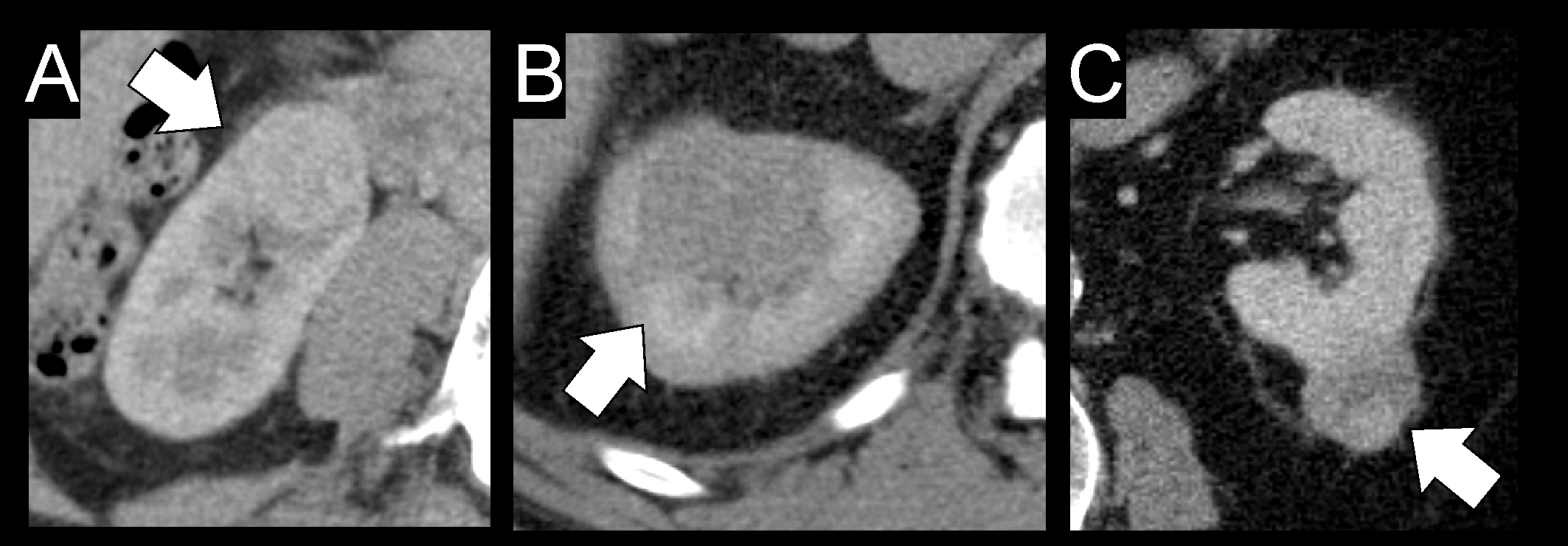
CT image examples of three different patients with renal cell carcinoma and different tumor nuclear grade. Whilst Fig 1A shows a grade 1 tumor of the right kidney of a 49 year-old female patient, Fig 1B is displaying a grade 2 clear-cell RCC of a 58 year-old male patient, and Fig 1C is from a 78 year-old male patient with a grade 3 tumor. All tumors are approximately the same size; however, a grading based on visual criteria only is not possible.
